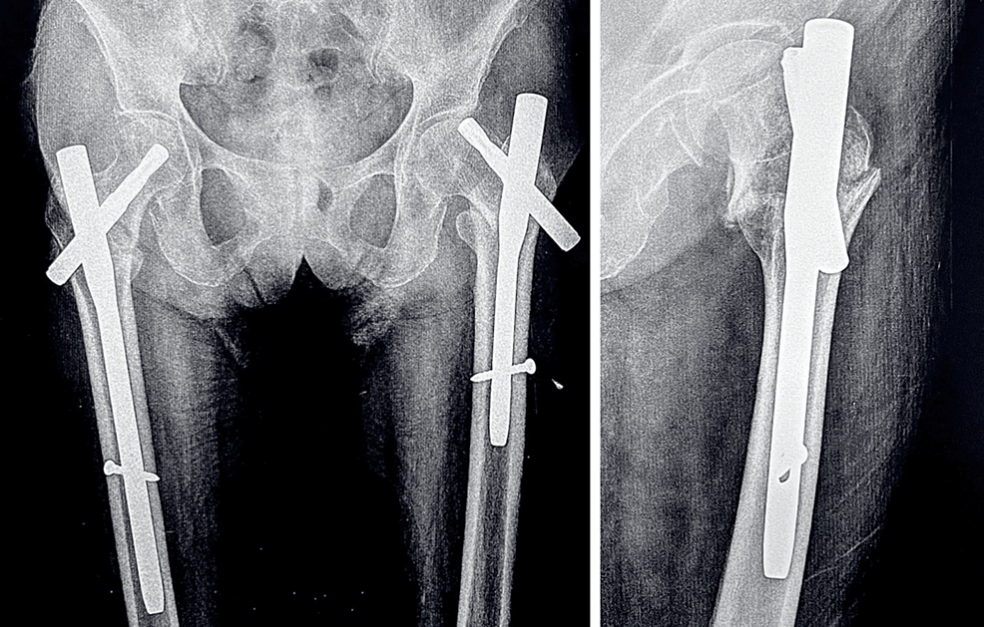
Plain radiograph of pelvis with both hips showing screw cut-out on the left side (postoperative day 15).
